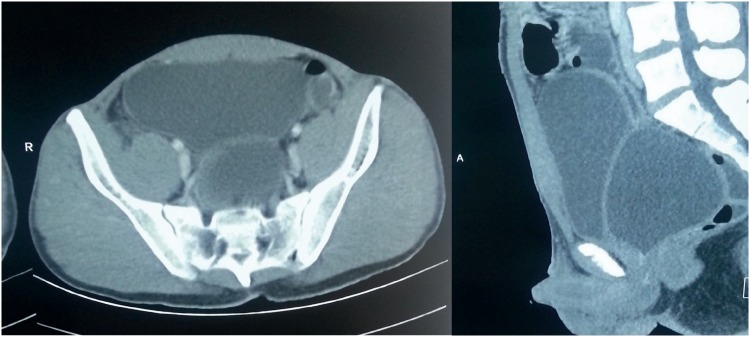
Preoperative CT scan showing the bladder anteriorly and prostatic utricle cyst posteriorly.
